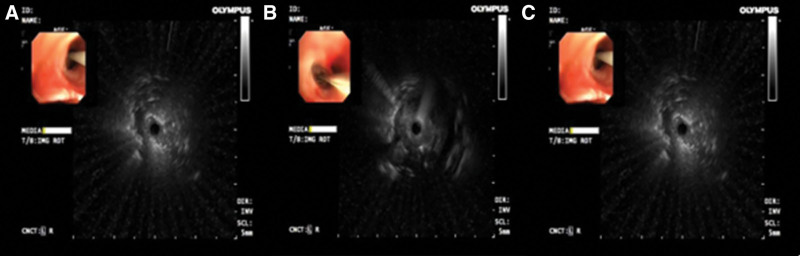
Radial endobronchial ultrasound-guided-transbronchial cryobiopsy showing mixed blizzard sign. (A) Lateral middle lobe of right lung; (B) anterior basal segment of right lower lobe; (C) external basal segment of right lower lobe.
